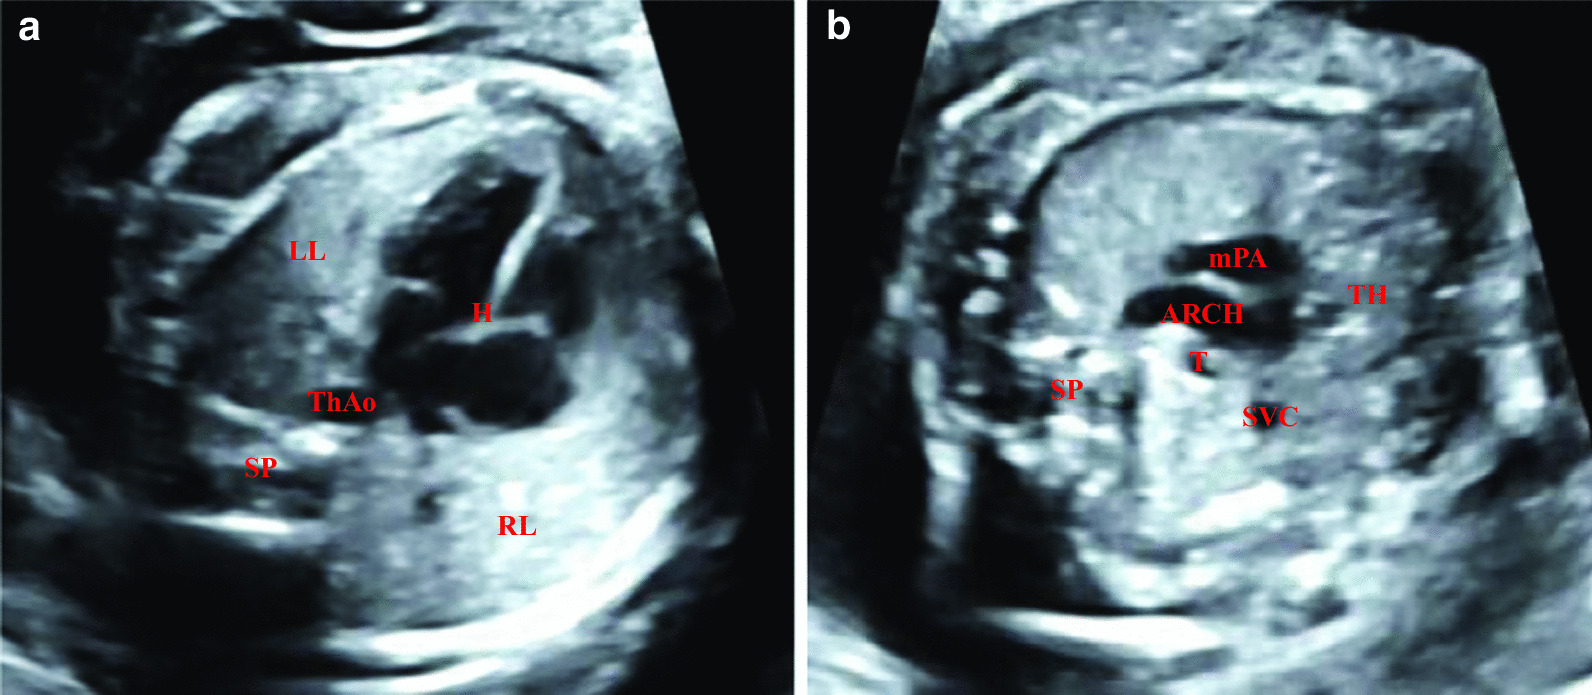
Normal ultrasonography of fetal thorax. a normal ultrasonography of fetal heart four-chamber view section; b normal ultrasonography of fetal three vessels trachea view section. H: heart, ThAO: thoracic aorta, SP: spine, LL: left lung, RL: right lung, SVC: superior vena cava, mPA: main pulmonary artery, ARCH: aortic arch, T: trachea, TH: thymus
